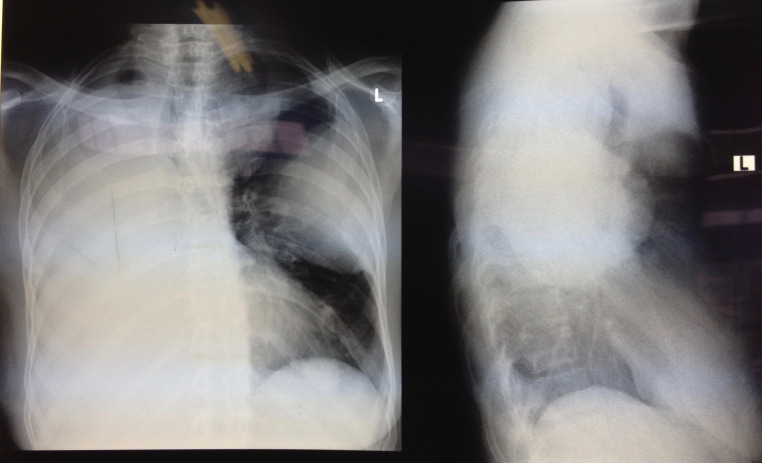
Posteroanterior and lateral plain films of Mrs AM showing nearcomplete white-out of the right hemithorax with tracheal deviation to
